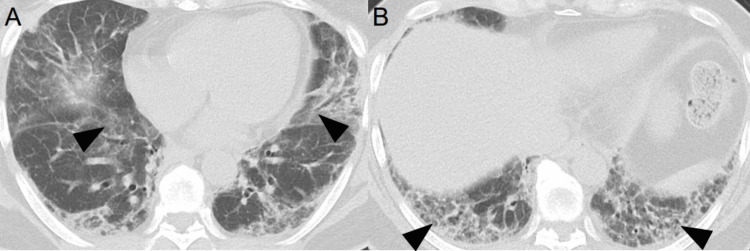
Plain chest computed tomography scan on admission showing A) frosted shadows (arrowhead) and consolidation and B) reticular shadows on the dorsal surfaces of the bilateral lower lung fields (arrowhead)
